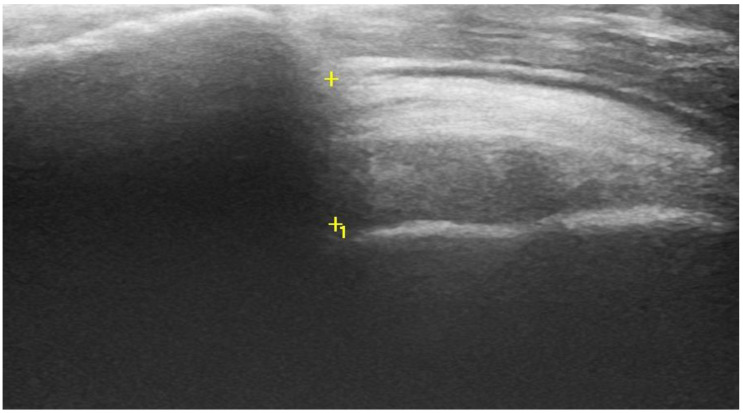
Example of ultrasonographic image of the acromio-humeral distance (AHD) at 0° of abduction. The measurement was the distance between the +/+1 marks.
